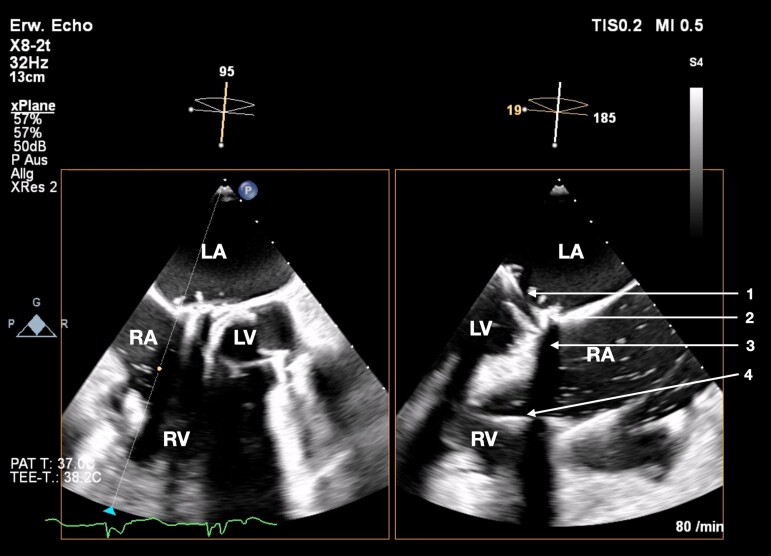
Transoesophageal echocardiography prior to EVOQUE implantation shows the transoesophageal echocardiography prior to implantation. Left: mid- to deep-oesophageal right ventricle inflow–outflow view; right: corresponding biplane view/inverted four-chamber view. The acoustic shadow from the aortic valve bioprosthesis and mitral valve reconstruction impairs the view. 1—mitral valve annuloplasty; 2—aortic valve bioprosthesis; 3—acoustic shadow; 4—implantable cardioverter-defibrillator lead (LA, left atrium; RA, right atrium; LV, left ventricle; RV, right ventricle).
